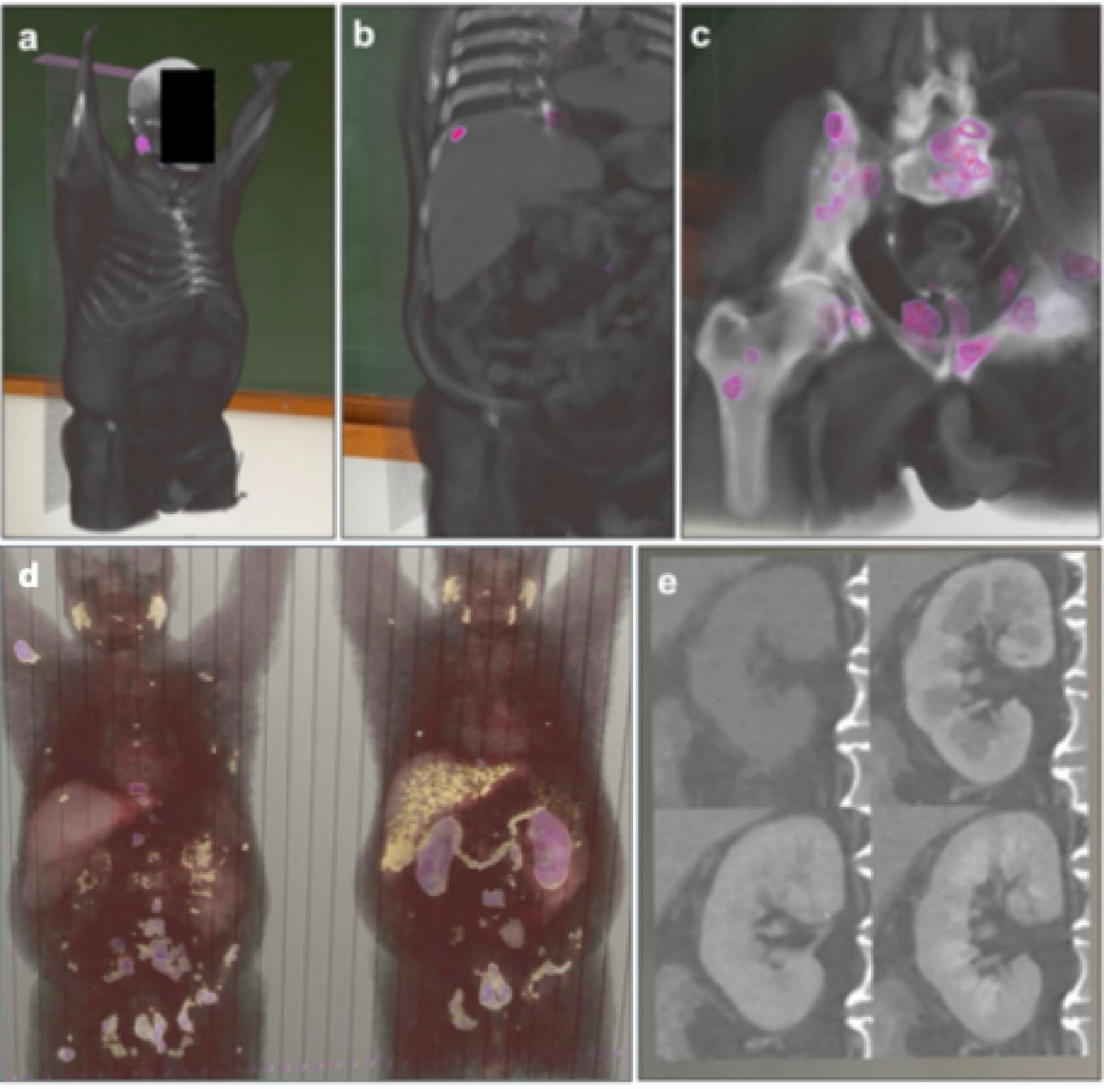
Integration of PET-CT and different time points using VR-prep. (a) AR images depict the entire scan volume with anonymized facial characteristics, (b-c) real-time cropping of the AR volume exposing a liver metastasis (b) and the pelvic tumor load (c). (d-e) Combination of scans from different time points in the same AR object. (d) PSMA PET patient before (left) and after (right) treatment show reduction of metastases. (e) right kidney of one patient at different time points: no contrast media (top left), arterial (top right), portal venous (bottom left) and venous (bottom right) phase of the contrast media passage.
